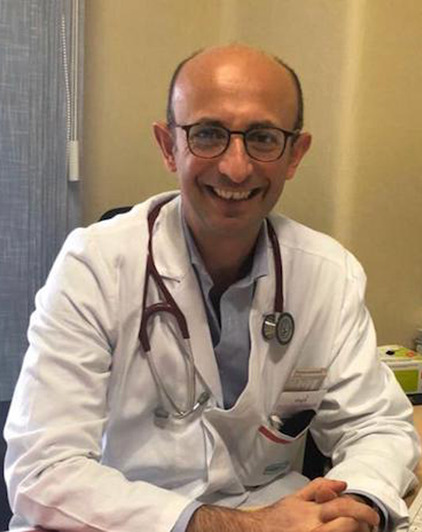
Dr Giancarlo Scognamiglio, MD, PhD, is a Consultant Cardiologist and Lead of the Imaging Programme in Adult Congenital Heart Disease at the GUCH Unit of Monaldi Hospital in Naples, Italy. During his training, he specialized in transthoracic and transoesophageal echocardiography and congenital heart disease. His fields of interest include congenital heart disease, valvular heart disease, pulmonary hypertension, ICU, transthoracic and transoesophageal echocardiography, and 3D echo. He is author of chapters in 4 scientific books and more than 40 scientific papers on Peer-Reviewed Journals. Dr Scognamiglio is Professor of Congenital Heart Disease in the Specialty Training Programme in Cardiology at the University of Campania Luigi Vanvitelli.
